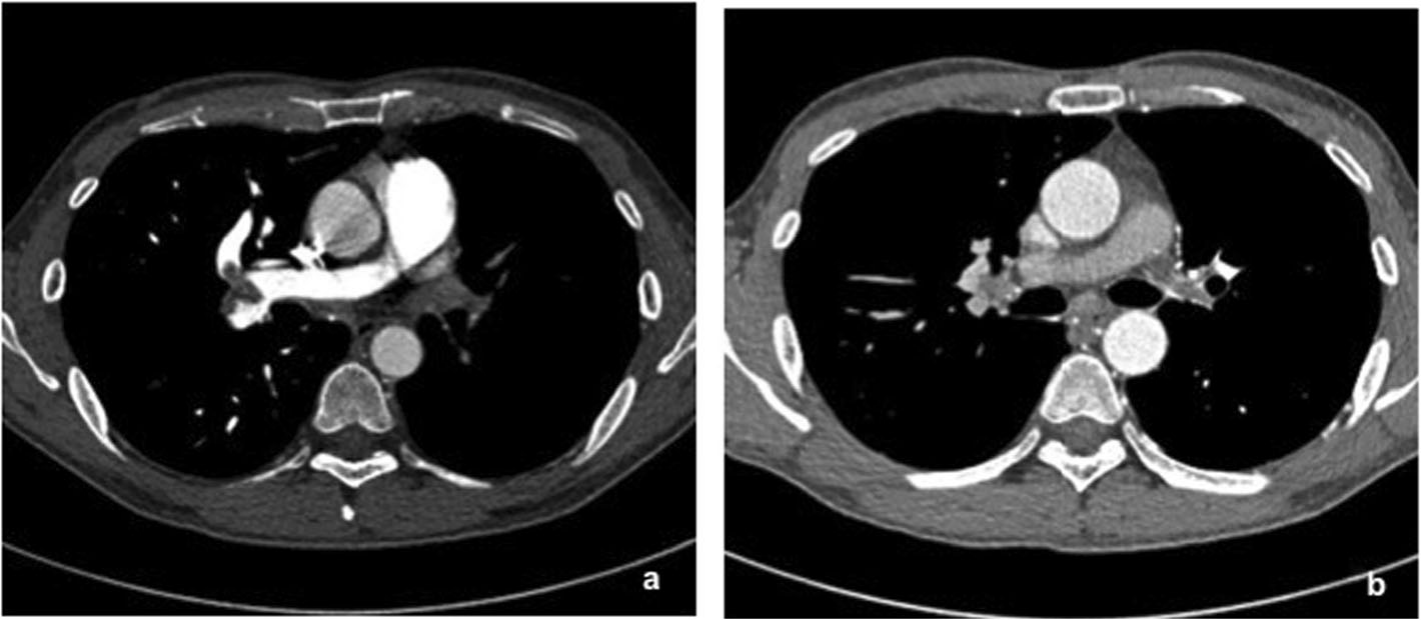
Thromboembolic disease of pulmonary artery on dynamic contrast-enhanced CT in a 44-year-old man. Axial CT revealed eccentric filling defects in the distal right pulmonary artery and its main branches suggesting acute pulmonary embolism(a). On the upper level, delayed CT revealed narrowing left pulmonary artery stump caused by chronic thromboembolic disease (b)
